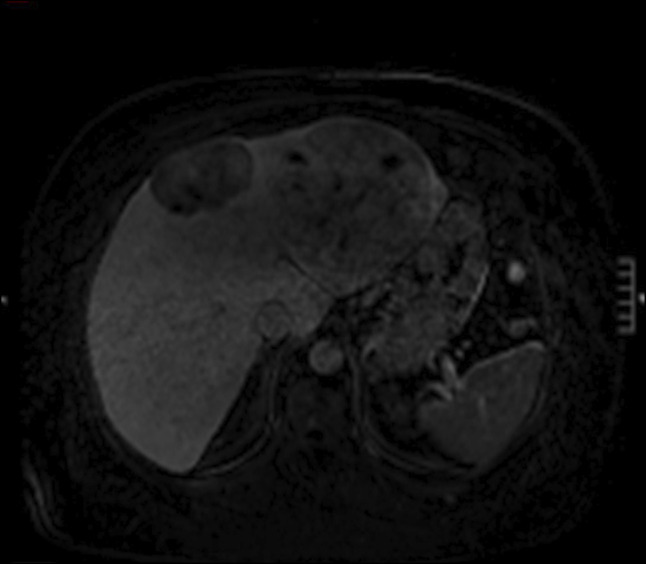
Magnetic resonance imaging shows multiple heterogeneously enhancing hepatic masses.
